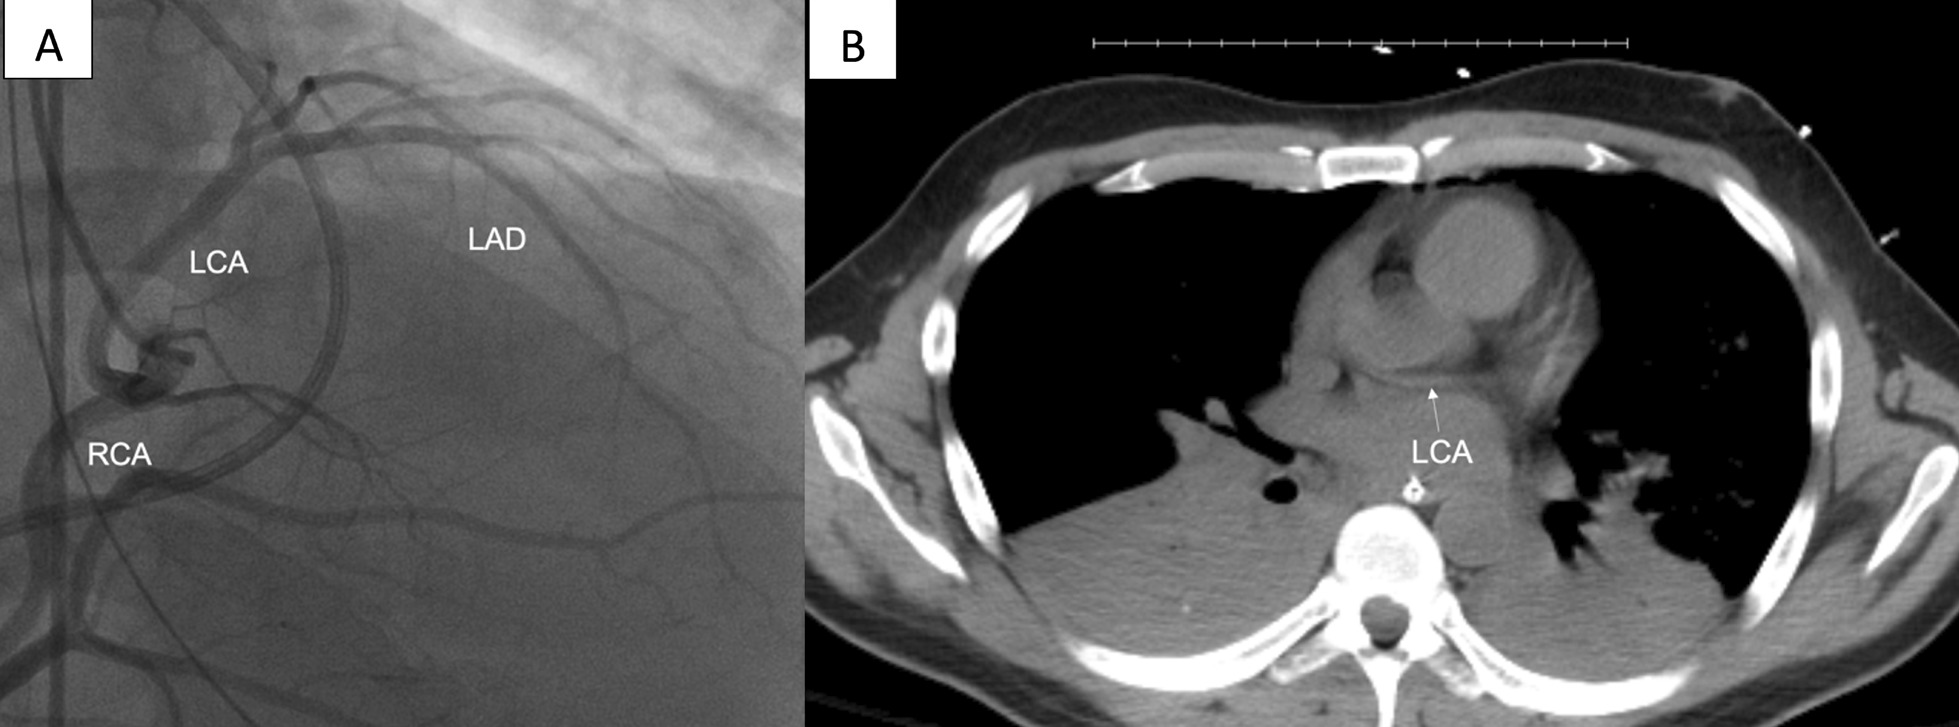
Evaluation of donor cardiac graft included A left heart angiogram demonstrating both left and right coronary arteries arising from single ostium within the right sinus of Valsalva and B chest computed tomography revealing retro-aortic course of the left coronary artery. *LAD* left anterior descending, *LCA* left coronary artery, *RCA* right coronary artery
